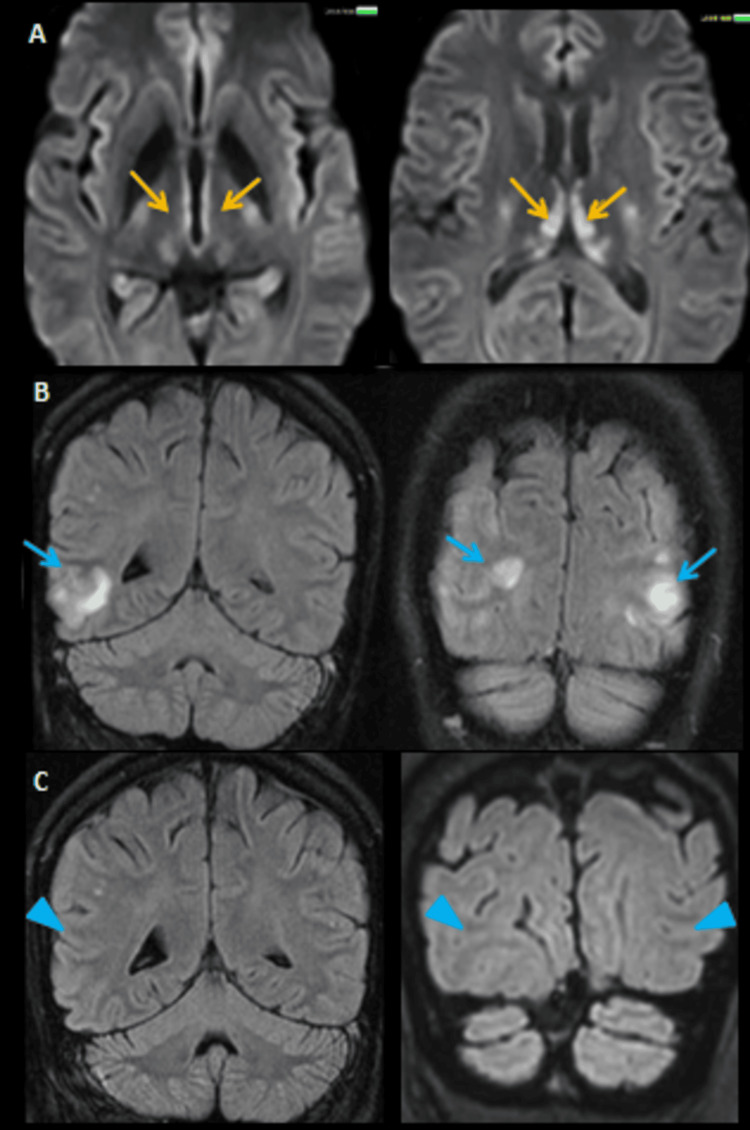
MRI findings in a 76-year-old male with altered sensorium and a history of hypertension, diabetes mellitus, and chronic kidney disease on long-term hemodialysis. (A) Axial DWI sequences show symmetrical hyperintensities around the third ventricle, dorsomedial thalami, and periaqueductal gray matter (orange arrows), suggestive of WE. (B) Axial and coronal FLAIR images reveal bilateral parieto-occipital cortical and subcortical hyperintensities (blue arrows). (C) Follow-up imaging shows resolution of parieto-occipital lobe signal changes on FLAIR sequence, correlating with clinical improvement (blue arrowhead). The imaging features are consistent with dysmetabolic encephalopathy due to a combination of WE and dialysis-related metabolic stress MRI: magnetic resonance imaging, DWI: diffusion-weighted imaging, WE: Wernicke’s encephalopathy, FLAIR: fluid attenuated inversion recovery
